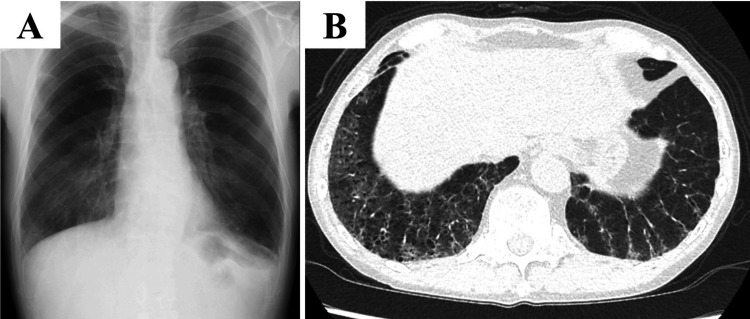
Chest X-ray and chest high-resolution computed tomography at a year after diagnosis A: On the chest X-ray, ground-glass opacity was improved. B: Chest high-resolution computed tomography, ground-glass opacity with interlobular septal was improved.
